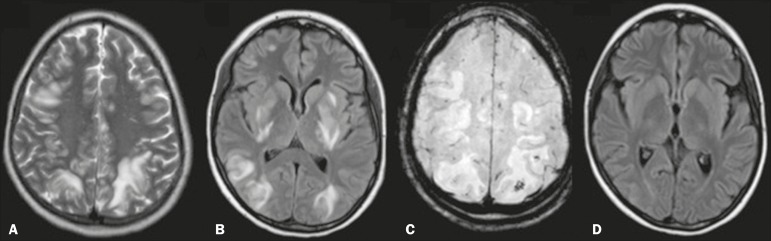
MRI scan of the skull of a female patient diagnosed with systemic lupus
